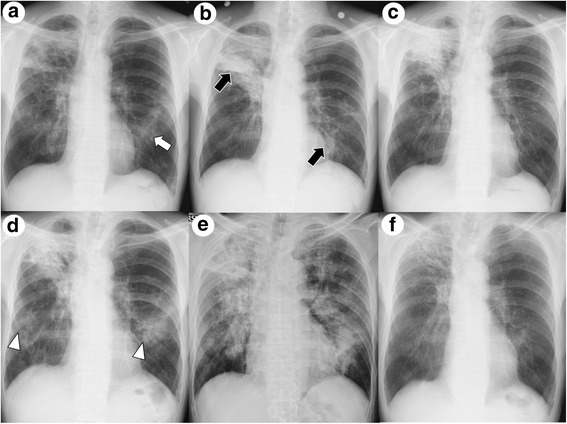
Chest imaging performed during the observation. a At 6 months after the referral. New infiltrates appeared in the left middle field (white arrow). b At 14 months after the referral. The consolidation in the right upper field was deteriorated, and the infiltrates in the left middle field migrated toward the lower right (black arrows). c At 25 months after the referral. The infiltrations in the left lung field were improved without treatment. d At 28 months after the referral. New infiltrates appeared bilaterally (white arrowhead) e At 38 months after the referral. The patients developed apparent aspiration pneumonia, with chest radiograph showing bilateral consolidations. f At 5 years after treatment against *M. fortuitum* was started. Multiple pulmonary lesions improved
